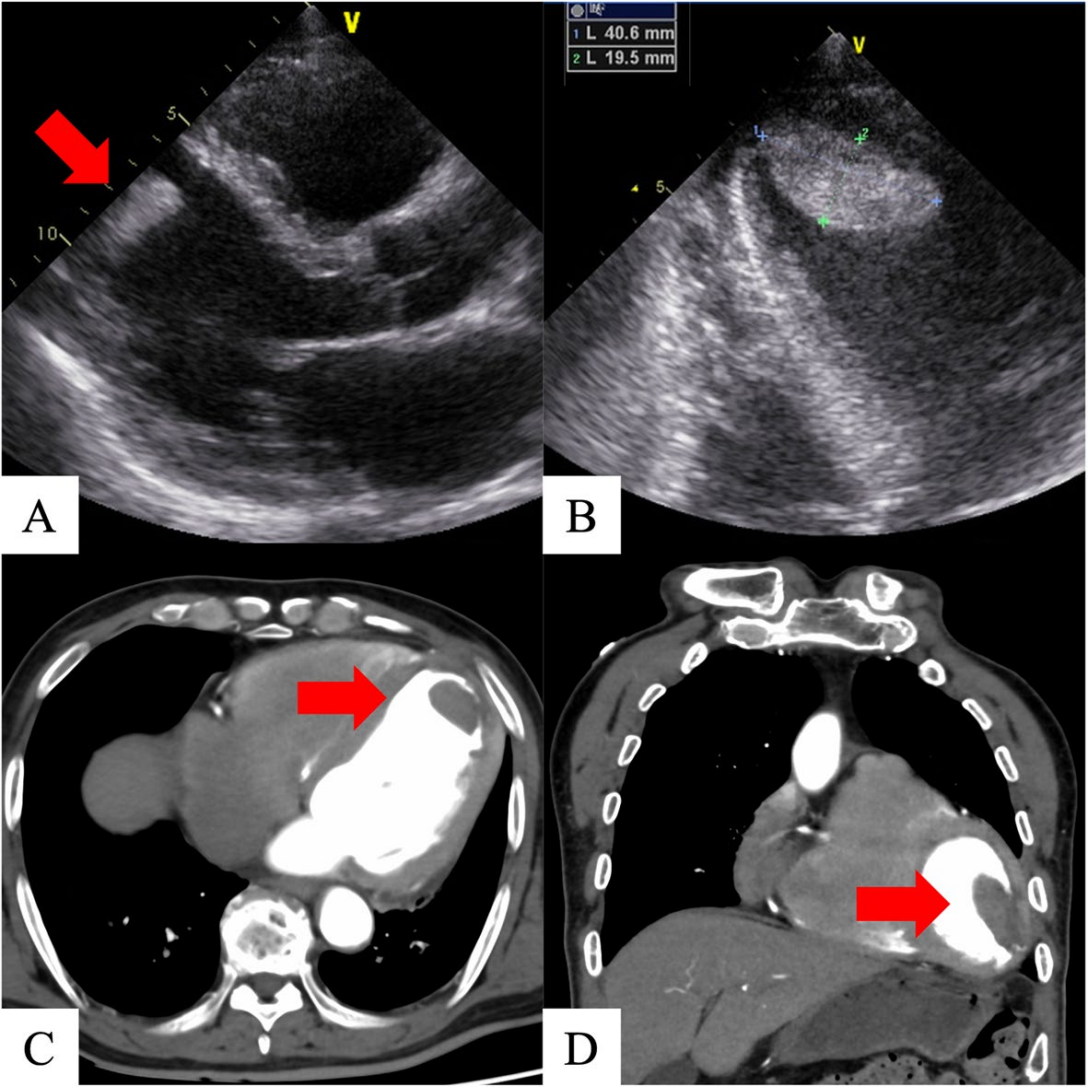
Preoperative transthoracic echocardiography and computed tomography. Transthoracic echocardiography shows anteroseptal and apical wall motion with akinesis and an apical thrombus (A). Transthoracic echocardiography shows a massive apical thrombus (40.6 × 19.5 mm) (B). Three-dimensional computerized tomography also shows the apical thrombus (C, D)
